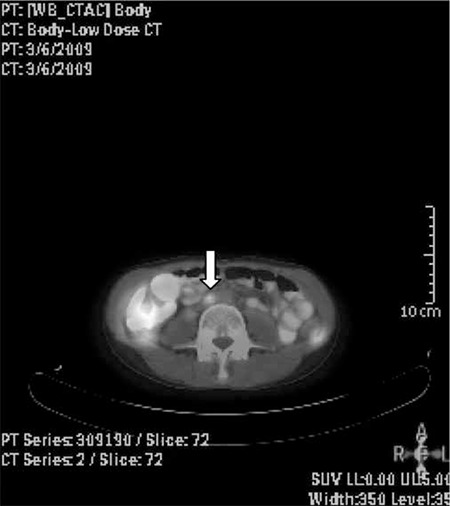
Paraaortic metastatic lymph node (arrow) detected by PET/CT in a patient who was suspected of having recurrence because of increase in tumor markers without definite findings on prior imaging modalities.
